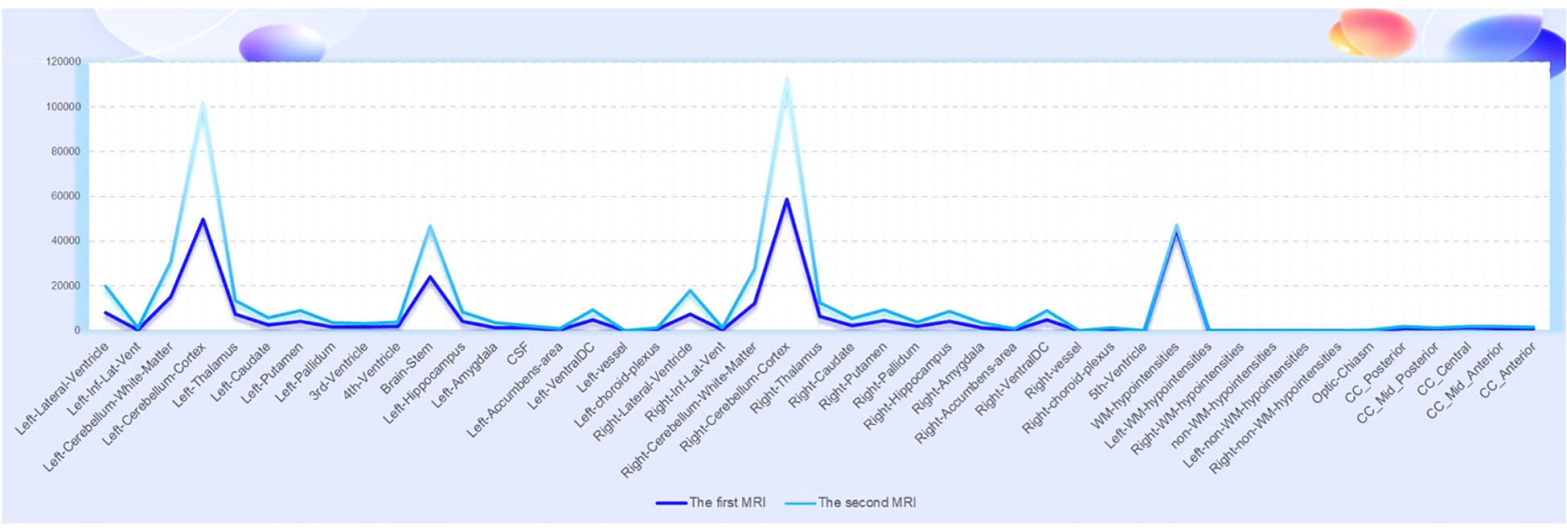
The comparison of the imaging findings between the first MRI (healthy state) and the second MRI (ARFID state) revealed an increase in the volume of some brain regions and nuclei.
